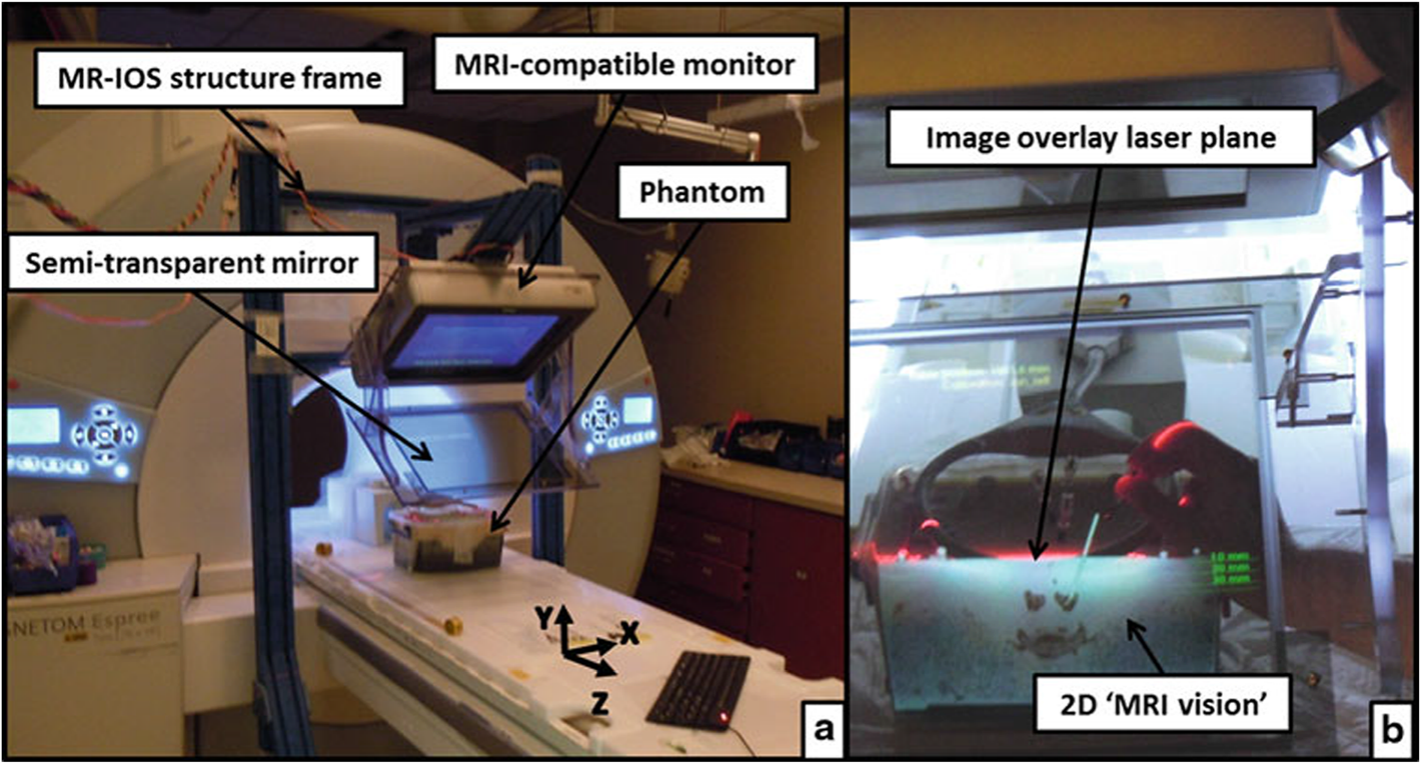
Reprinted by permission from Springer Nature: Springer, International Journal of Computer Assisted Radiology and Surgery, MR image overlay guidance: system evaluation for preclinical use, Paweena U-Thainual, Jan Fritz, Choladawan Moonjaita et al., Copyright 2012. a) Visualized is the MR-IOS. b) The surgeon looks through the semi-transparent mirror that is augmented with the correct insertion path
